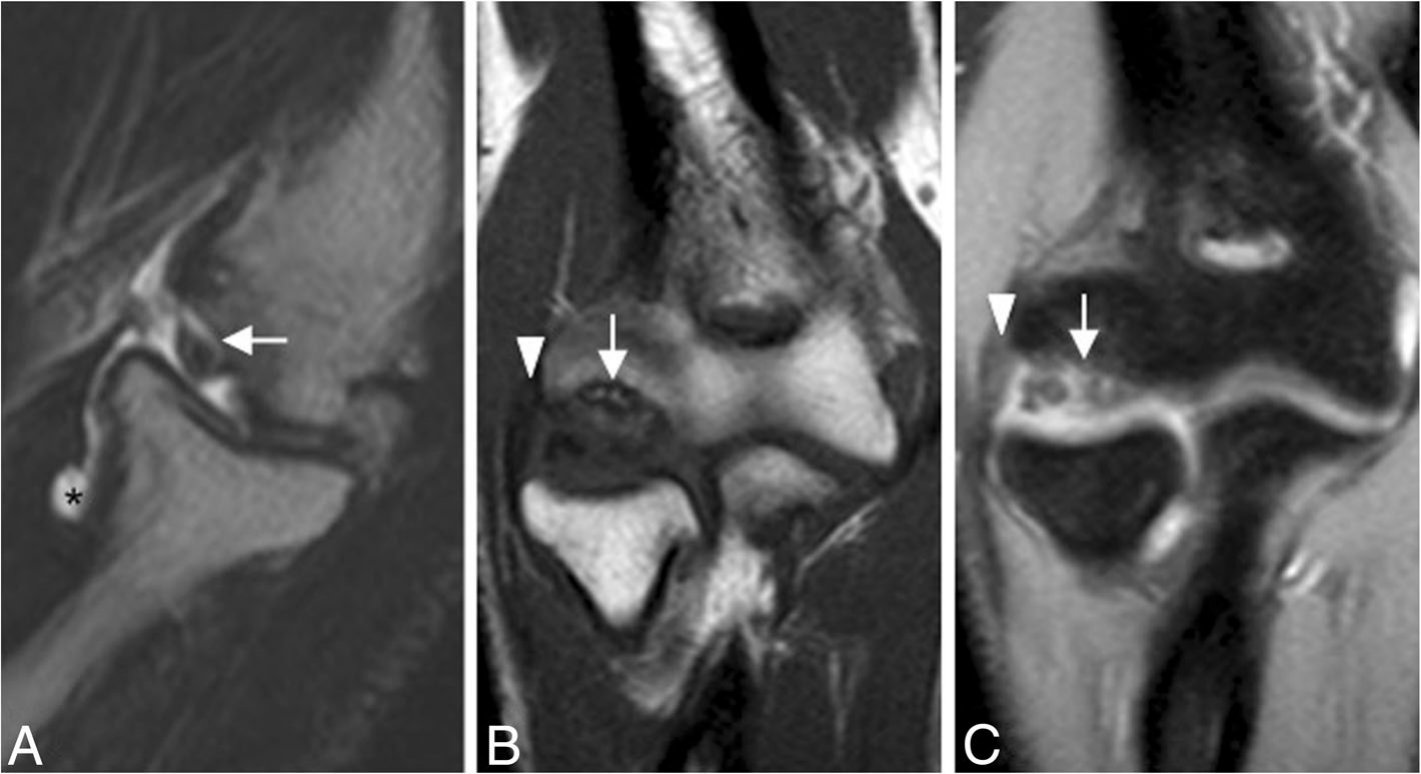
A 33-year-old female gymnast with elbow pain and instability. Sagittal T2-weighted MRI (a), coronal T1-weighted MRI (b), and coronal FS PD-weighted MRI (c), demonstrating an osteochondral lesion in the posterior capitellum caused by posterolateral rotatory instability. The lesion is unstable, with fluid extending into the interface between the fragment and the parent bone (white arrows). Proximal partial disruption of the lateral ulnar collateral ligament (white arrowheads). Joint effusion (black asterisk)
